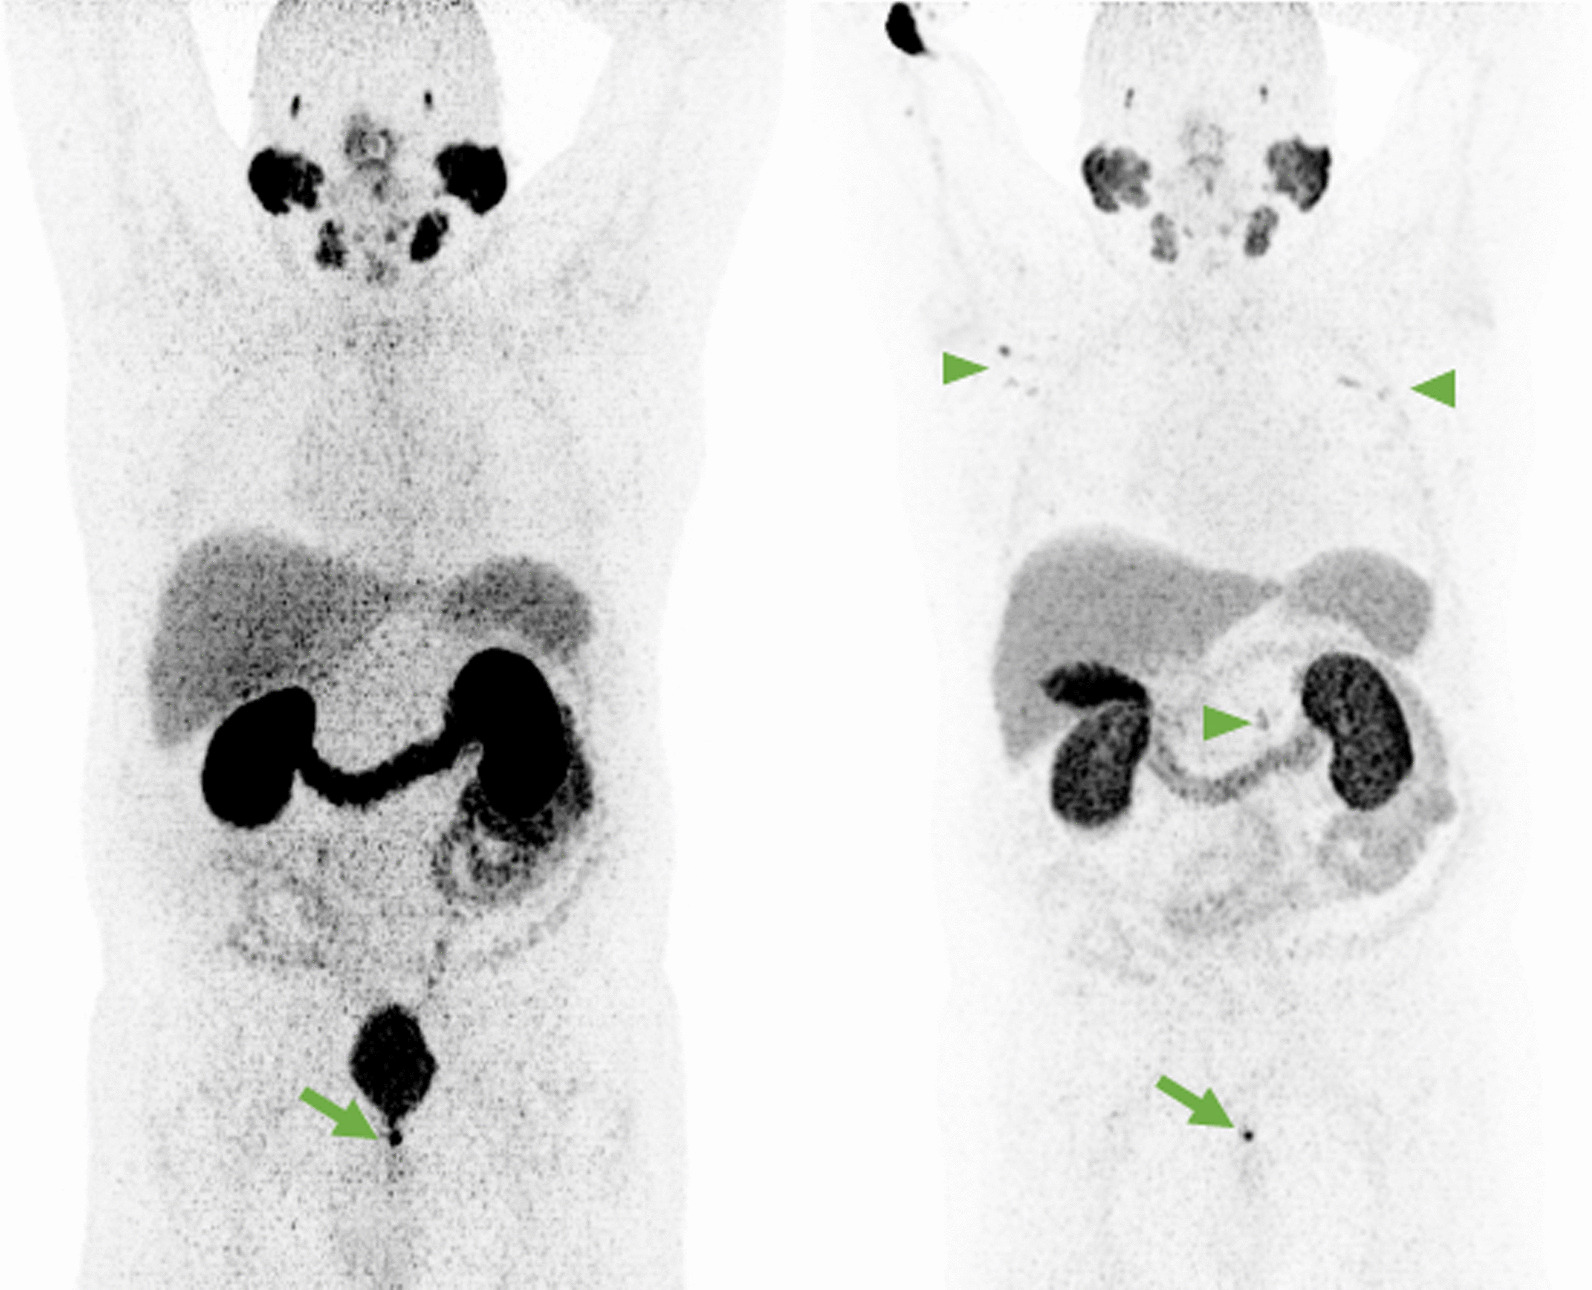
MIP of [^68^Ga]-Ga-PSMA-11 and [^18^F]-F-PSMA-1007 PET: Beside the concordant local recurrence in the left prostate bed (arrow), there is unspecific oligofocal uptake in the rip thorax, lymphonodally in the right axilla, the coeliac ganglia (arrow heads) and the gall bladder
